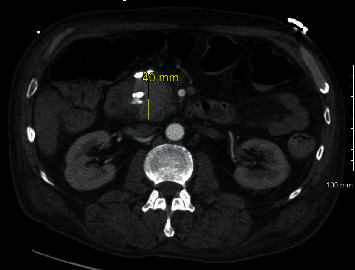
Computed tomography with angiography with measurement of pseudoaneurysm.
